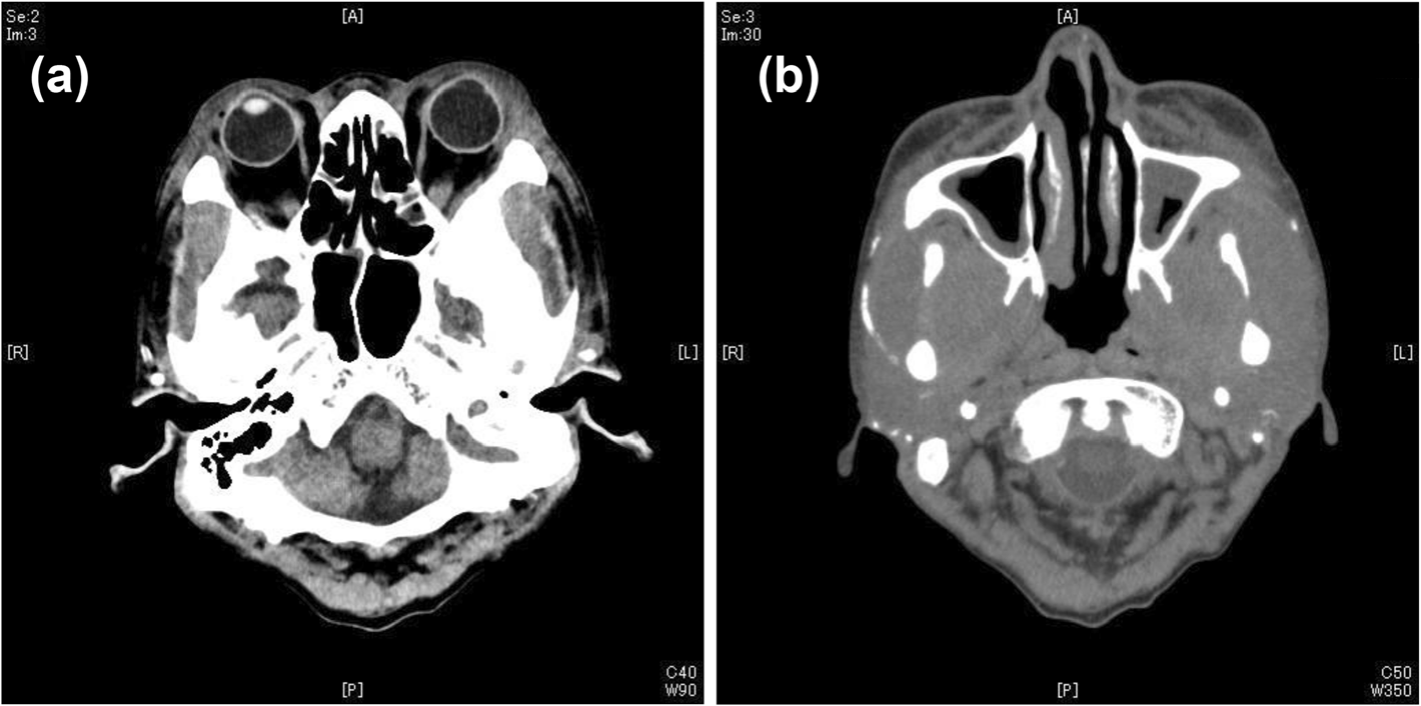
Computed tomography scan of the facial lesion about 1 month after the admission. Computed tomography shows findings consistent with (a) diffuse osteosclerotic change on orbits and skull base and peri-orbit soft tissue swelling, (b) mucous thickening of maxillary sinus and marked swelling of masseter.
